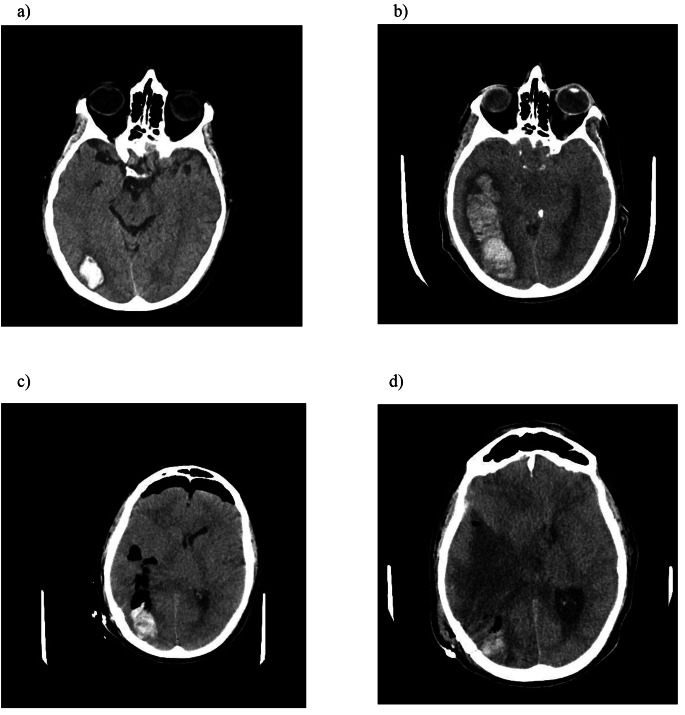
Eevolution of intraparenchymal hematoma in the temporo-parietal area: a) First CT performed; b) Second CT scan performed – hematoma maximum antero-posterior lenght 9 cm; c) CT after surgical decompression; d) Last CT performed shows only a partial reduction in the shift of the median structures with blood component and air bubbles near the surgical brain bed
